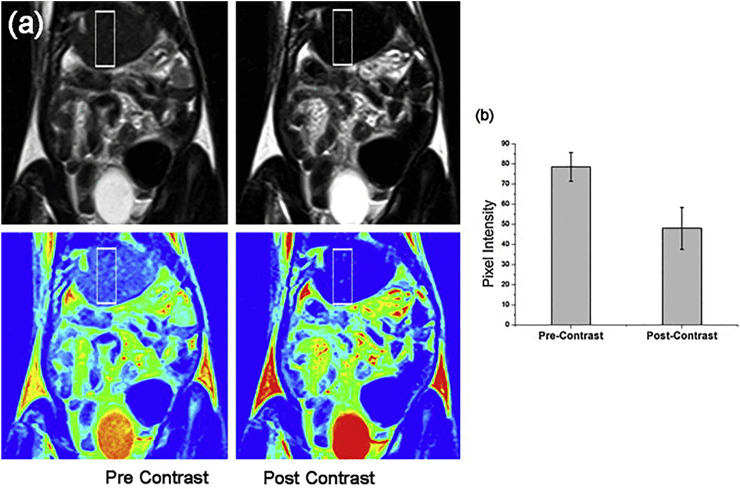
Comparison of pre- and post-contrast *in vivo* MRI of the liver in a region of interest from fibrosed areas (above) and in colorized images of the original MRI (below) after 10 min of application of citrate-coated ultrasmall SPIONs (A). The graph of pixel intensity in pre- and post-contrast of liver is presented in (B). Reproduced with permission (Saraswathy et al., 2014) Copyright 2014, Elsevier B.V.
